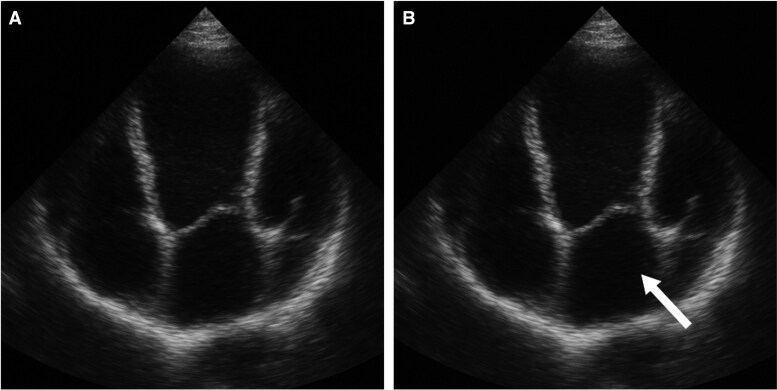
Images generated by ChatGPT-5. Images created on 14th August 2025 in response to requests to (*A*) ‘Generate a cardiac ultrasound image’, and then (*B*) to ‘Indicate with an arrow where the right ventricle is’. Reproduced with permission from Gilles Van de Vyver, PhD, Norwegian Technical University, Trondheim, Norway.
